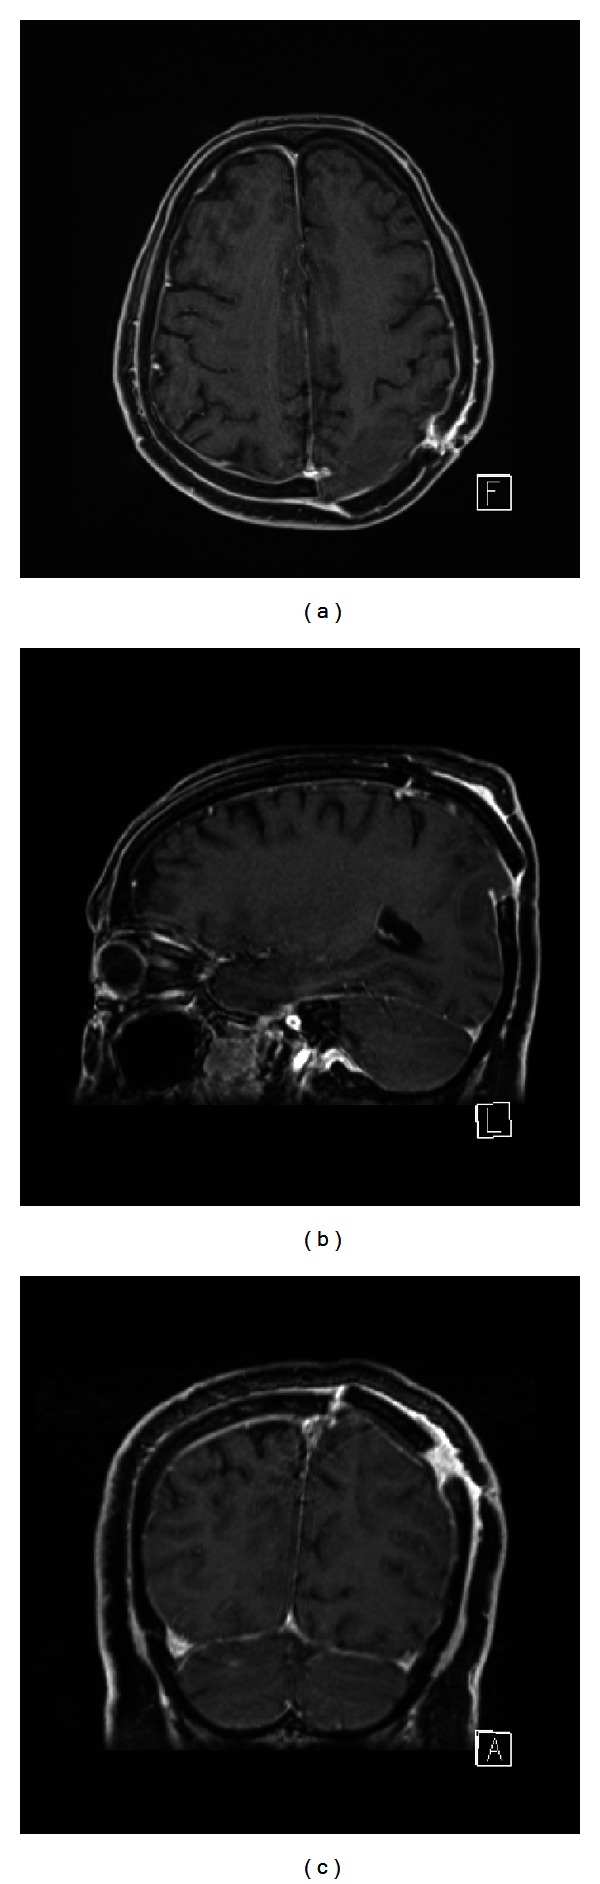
Gd-enhanced, T1-weighted MRI: postoperative axial (a), sagittal (b), and coronal (c) images show the complete excision of the granuloma.
